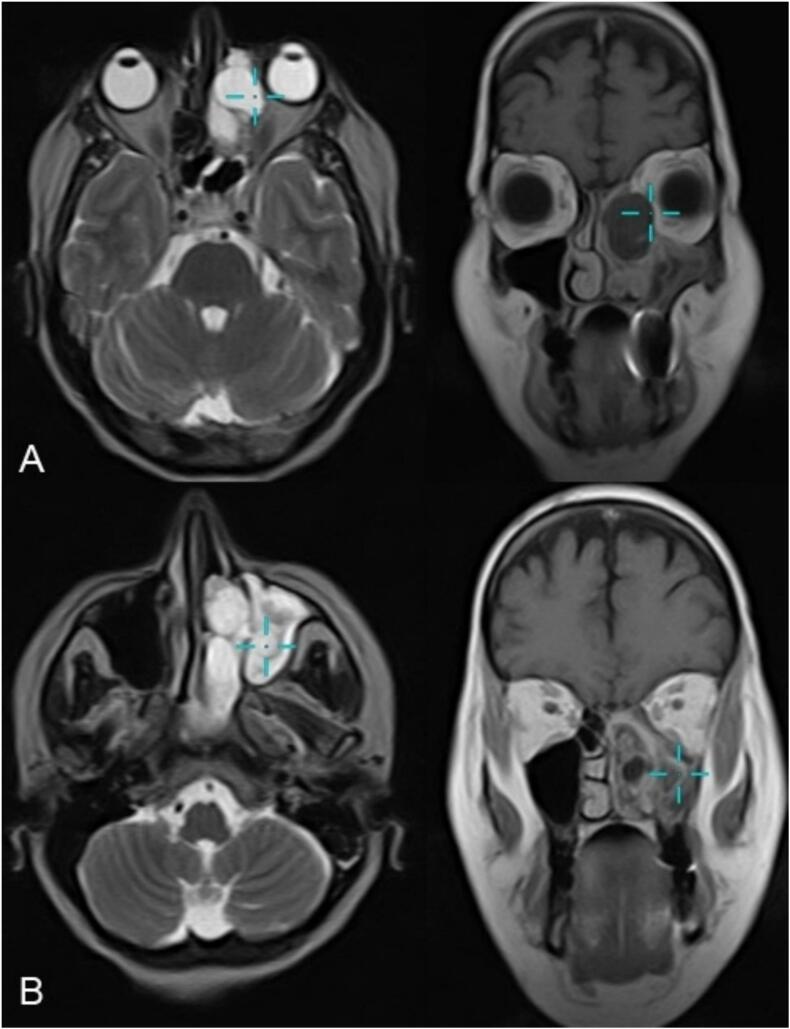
MRI images. A: Axial T2-weighted image showing a bright lesion impinging on the orbit. The corresponding coronal section with T1-weighted imaging post‑gadolinium contrast reveals a lesion that does not enhance. B: The lesion located within the maxillary sinus, also showing no uptake of gadolinium enhancement.
